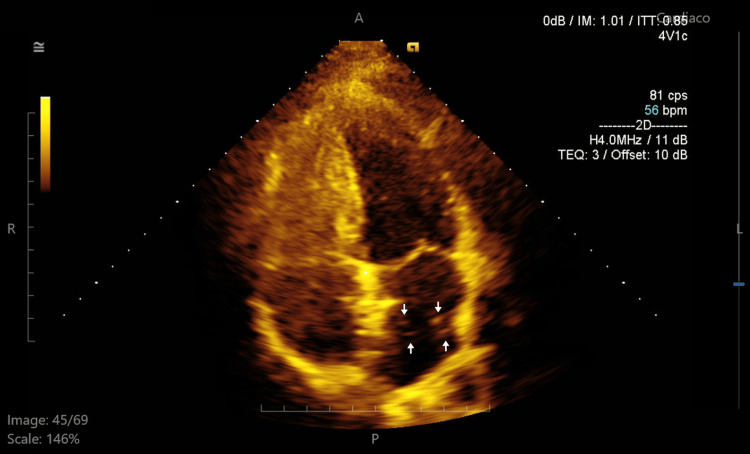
Four-chamber apical view in transthoracic echocardiography showing the passage of a few bubbles through the distal edge of the device.
